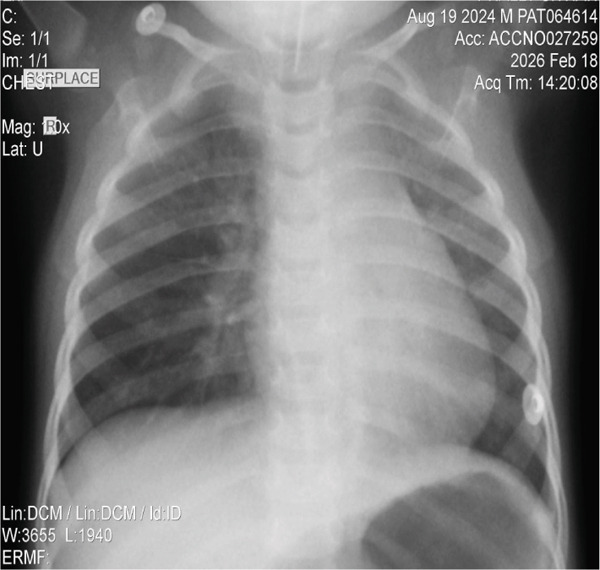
Follow‐up chest radiograph demonstrating re‐expansion of the left lung after bronchoscopic removal of mucus plugs.
